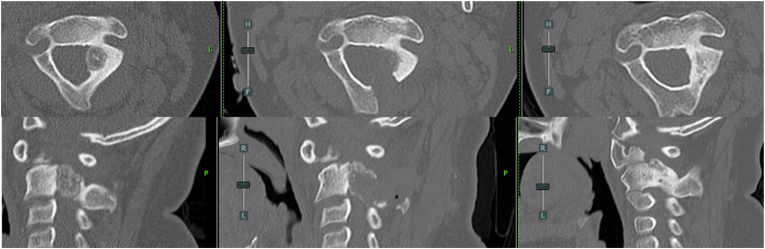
Comparative CT images (left to right): preoperative, immediate postoperative, and 3-year postoperative.
